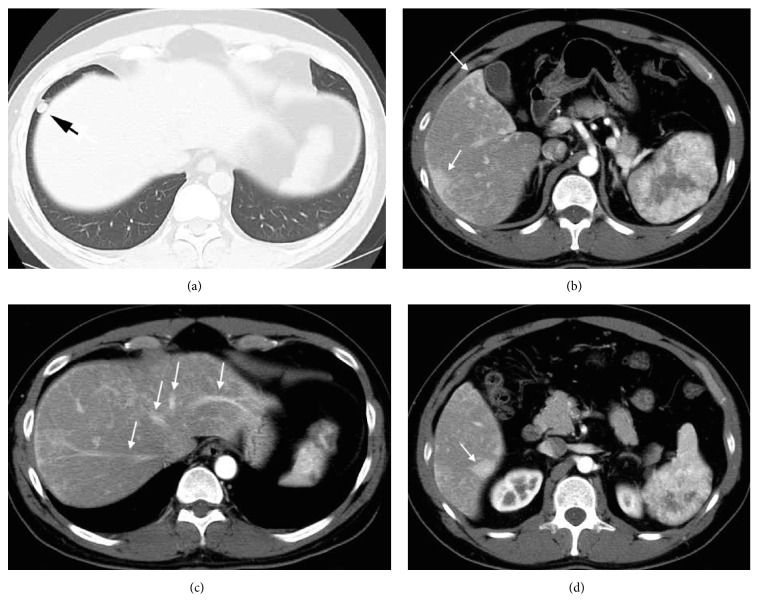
Arterial phase of enhanced CT, showing (a) a pulmonary arteriovenous malformation (arrow); (b) transient perfusion abnormalities (arrow) in the periphery of the liver; (c) three major hepatic veins (arrow), suggesting arteriosystemic shunts of the liver; (d) a large confluent vascular mass (arrow) in the periphery of the liver.
